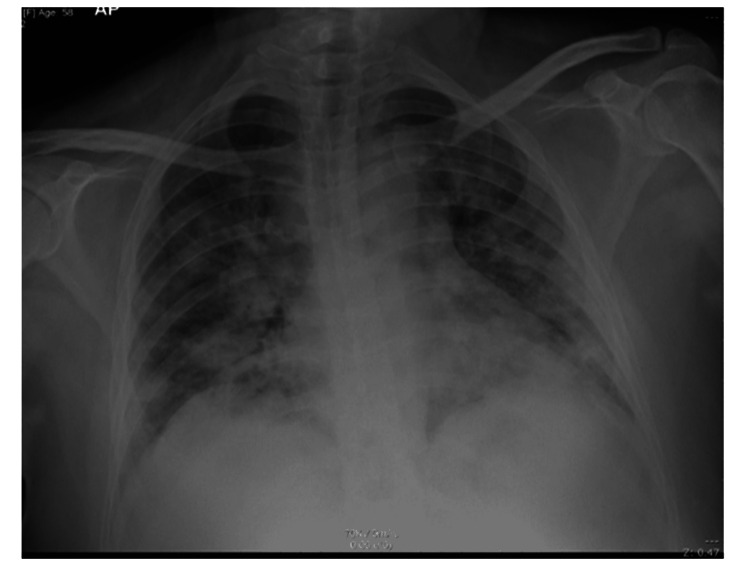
Frontal chest X-ray shows bilateral multiple confluent alveolar peripheral and medial peribronchovascular infiltrations with air bronchogram.
